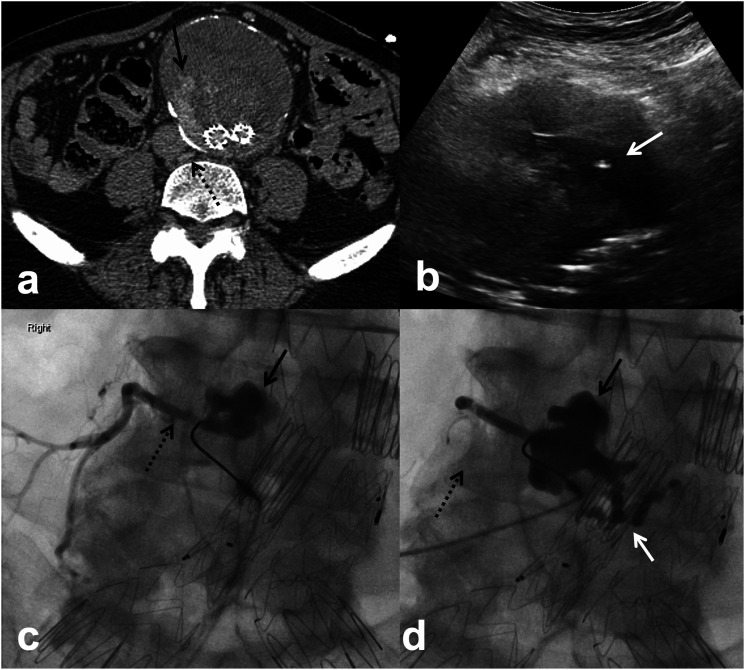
82-year-old man with previous EVAR in 2016 and transarterial coil embolization of the IMA for T2EL in 2017. Routine follow-up CTA in 2019 (a) demonstrated recurrent T2EL (solid arrow) which appeared to arise from a lumbar branch at L4 (dashed arrow). Direct sac puncture of the endoleak nidus was performed under US from a transabdominal approach (b) using a 22-G needle targeting the hypoechoic area within the sac (solid arrow). Initial angiography during contrast injection through the needle (c) demonstrates opacification of the endoleak nidus (solid arrow) and the right L4 lumbar artery (dashed arrow). The endoleak was subsequently embolized using 1:6 nBCA glue/Lipiodol mixture filling both the endoleak nidus (solid black arrow) and the right lumbar artery (dashed arrow). In addition, there was also filling of a left lumber artery (solid white arrow) not seen on initial angiography, suggesting that this may have been the inflow vessel. Follow-up CTA demonstrated aneurysm sac stability and resolution of endoleak at 2 years.
